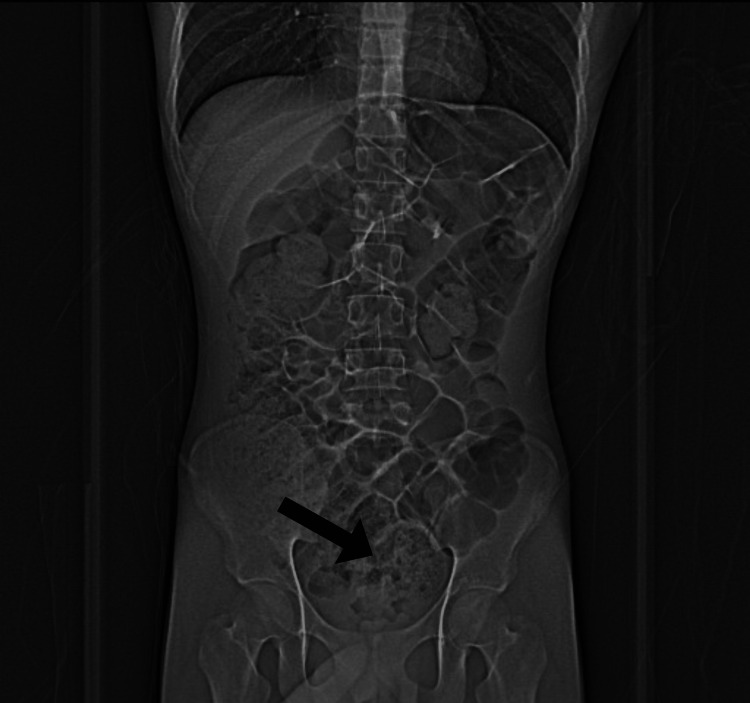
Abdominal computed tomography from outside institution. Heterogeneously enhancing soft tissue (indicated by black arrow) and fluid distending the rectum with very large colonic and rectal stool volume were observed.
